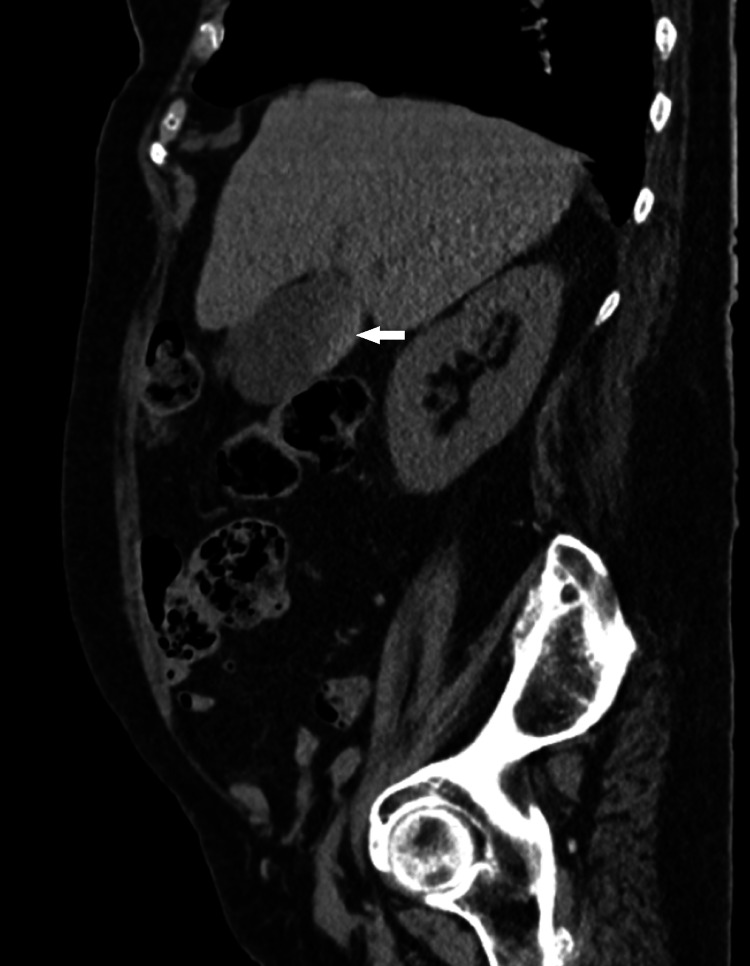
Intraluminal mass on pre-contrast thoraco-abdomino-pelvic computed tomography (TC scan) with no enhancement on post-contrast computed TC scan Arrow: Gallbladder lesion
